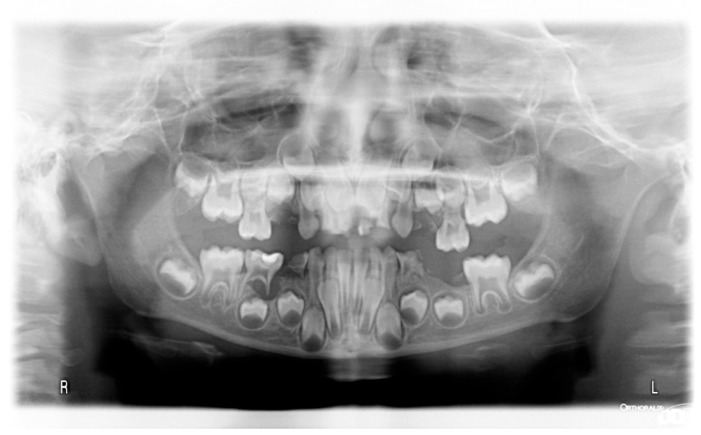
Orthopantomography X-ray before treatment (clinical case #2).
